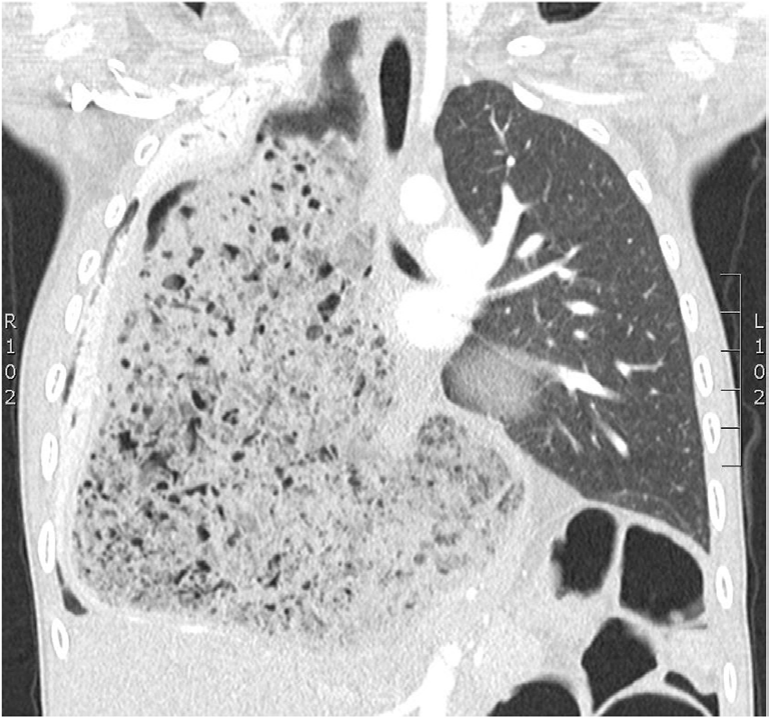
Coronal section of chest CT scan. Gross distension of the stomach and a giant bezoar containing gas and food debris causing unusual patterning in the right hemithorax.
